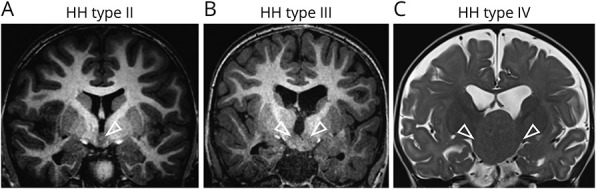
Representative MRI of HH Subtypes (A) MRI T1 coronal sequence of patient ICM_239 with HH type II attached to one side of the hypothalamus (white arrowhead). (B) MRI T1 coronal sequence of patient ICM_242 with HH type III attached bilaterally to the hypothalamus (white arrowheads). (C) MRI T2 coronal sequence of patient ICM_212 with a giant HH type IV with bilateral attachment to the hypothalamus (white arrowheads) and ventricular enlargement.
